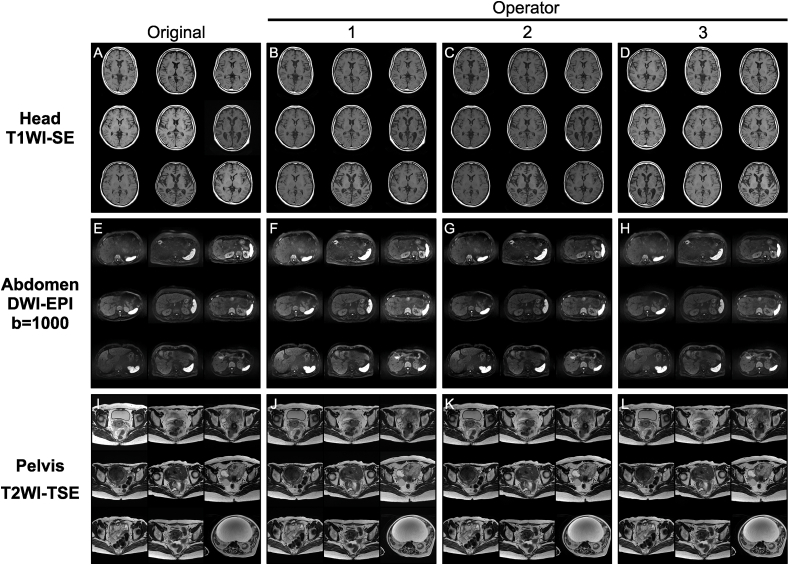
Representative magnetic resonance (MR) images of the test set displayed with the original window level and width (WL/WW; A, E, I) and the predicted optimal WL/WW for each MRI operator (B–D, F–H, J–L). The acquisition sequence and body region are shown for brain spin echo T1-weighted image (T1WI-SE) in the first row (A–D), abdomen diffusion-weighted image with echo planer imaging (DWI-EPI) with b = 1000 in the second row (E–H), and pelvis turbo spin echo T2-weighted image (T2WI-TSE) in the third row (I–L), respectively.
